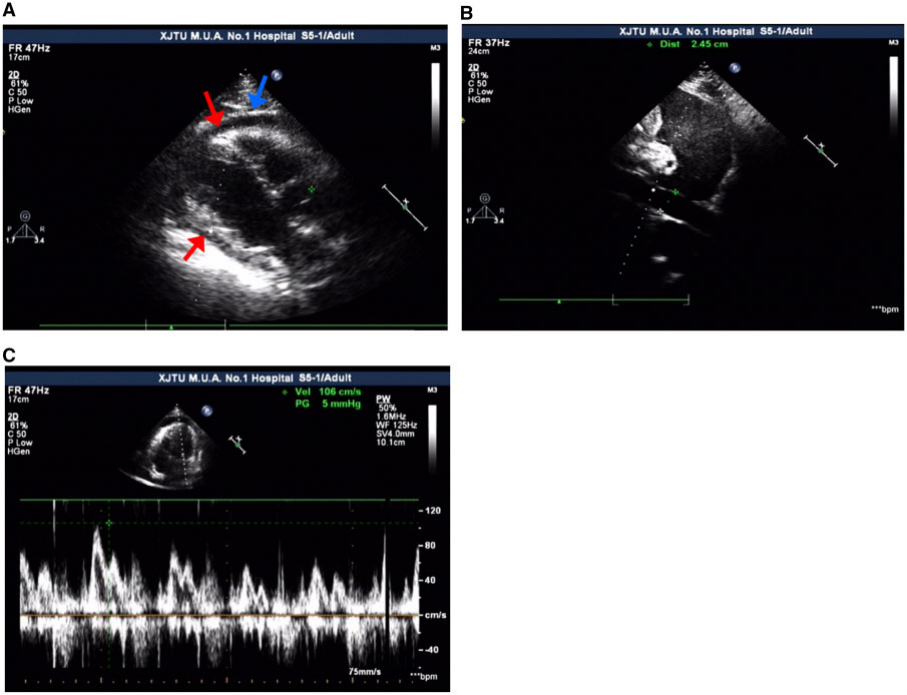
Echocardiogram showed (*A*) small-medium pericardial effusion (red arrows) with the data of left ventricular posterior wall 6.4 mm, right ventricular anterior wall 7.7 mm, apex of heart 6 mm, right ventricular free wall 17 mm, left ventricular free wall 6 mm, and thickened parietal pericardium (blue arrow); however, which was not observed by the sonographer. (*B*) Dilation of the inferior vena cava (24 mm). (*C*) Respiratory variation of the mitral peak E velocity of >25%.
